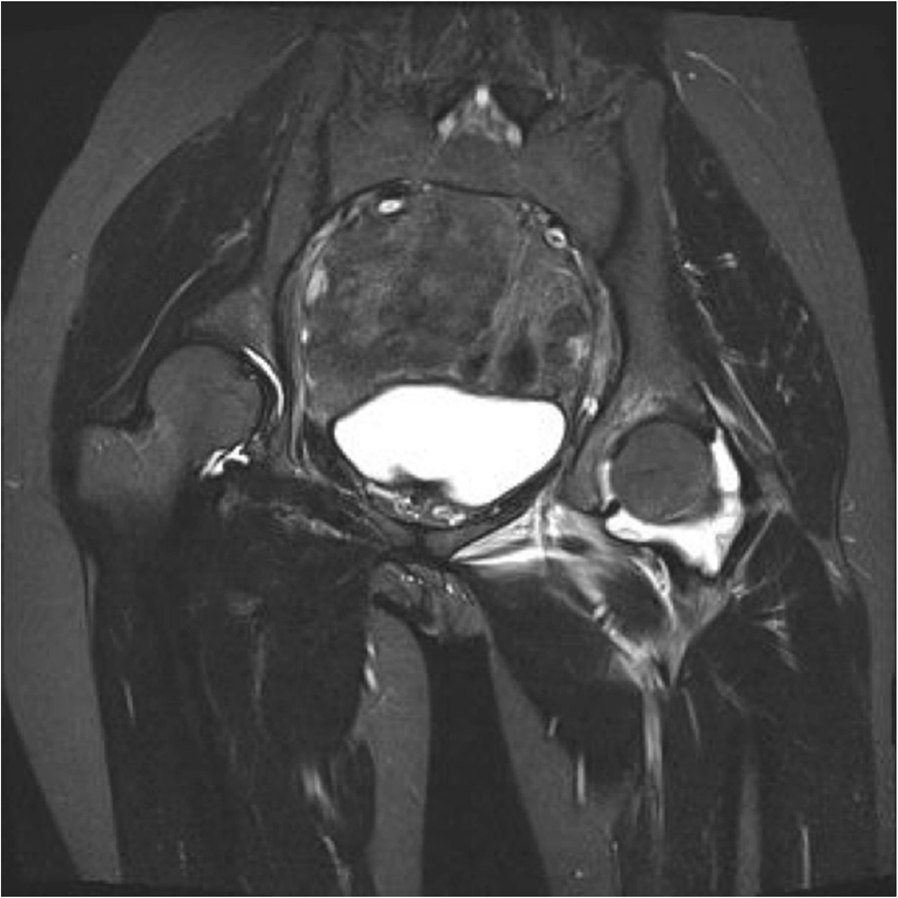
T2-weighted magnetic resonance imaging coronal plane of pelvis showed marked effusion over left hip joint.
